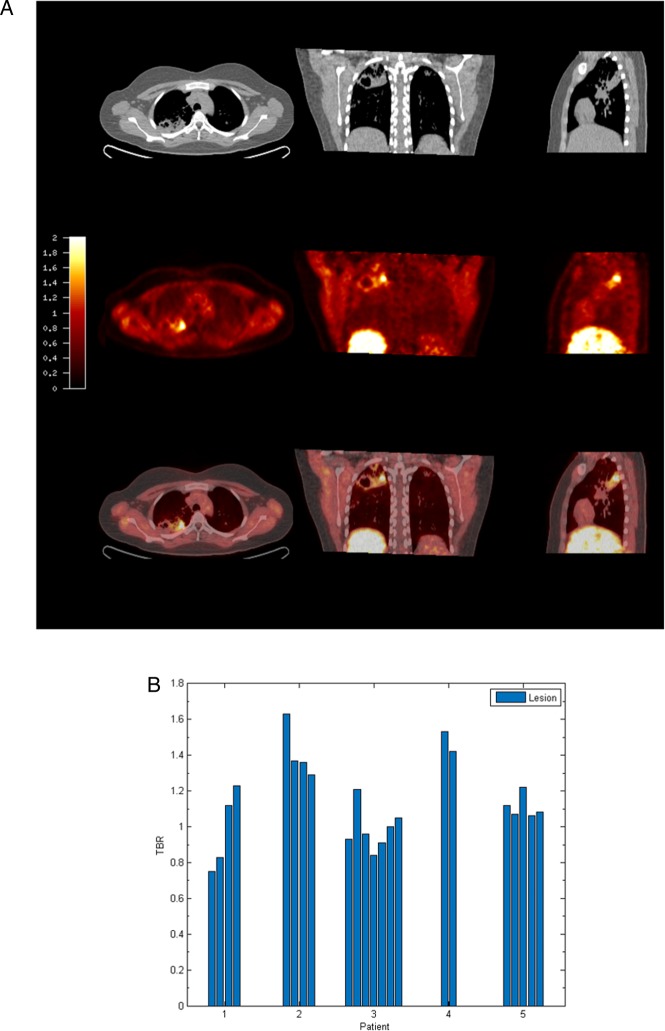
[^18^F] fluoromisonidazole ([^18^F]FMISO) PET-CT demonstrates increased tracer uptake within TB lesions. (A) [^18^F]FMISO PET-CT images. Transverse, coronal and sagittal slices through the [^18^F]FMISO target-to-background (TBR) and CT images of Patient 2. Top row, CT images show consolidation and a pulmonary cavity in the right upper lobe with a smaller, non-cavitating lesion in the left lung. Middle row, [^18^F]FMISO TBR map demonstrates intense uptake of tracer in the right upper lobe lesion and the liver, the site of metabolism of [^18^F]FMISO. Bottom row, coregistered CT and TBR images. (B) TBR values greater than unity were found for at least one lesion in all patients (each individual bar represents one region of interest, ROI).
